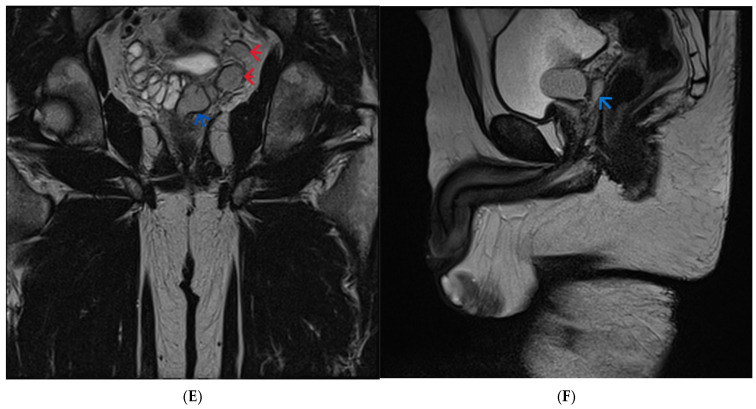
(A) sagittal T2-weighted MR image. Left ureterocele (orange arrow), enlarged left ureter (yellow arrow). (B) axial T2-weighted MR image. Left ureterocele (orange arrow), enlarged left ureter (yellow arrow). Seminal vesicle cysts (red arrows). (C) axial fat-suppressed T1-weighted MR image, with contrast. Left ureterocele (yellow arrow), dilated ejaculatory duct (blue arrow). (D) axial T2-weighted MR image showing: left ureterocele (yellow arrow), dilated ejaculatory duct (blue arrow). (E) coronal T2-weighted MR image showing: dilated ejaculatory duct (blue arrow) and seminal vesicle cysts (red arrows) (F) sagittal T2-weighted MR image showing: dilated ejaculatory duct (blue arrow).
